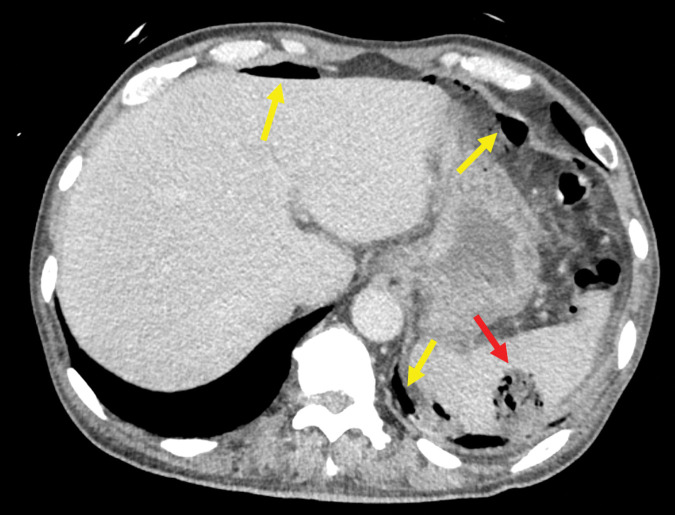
Contrast-enhanced computed tomography findings: A non-enhancing area containing gas was observed in the upper pole of the spleen (red arrow), along with free intraperitoneal gas that primarily surrounded the spleen (yellow arrow).
